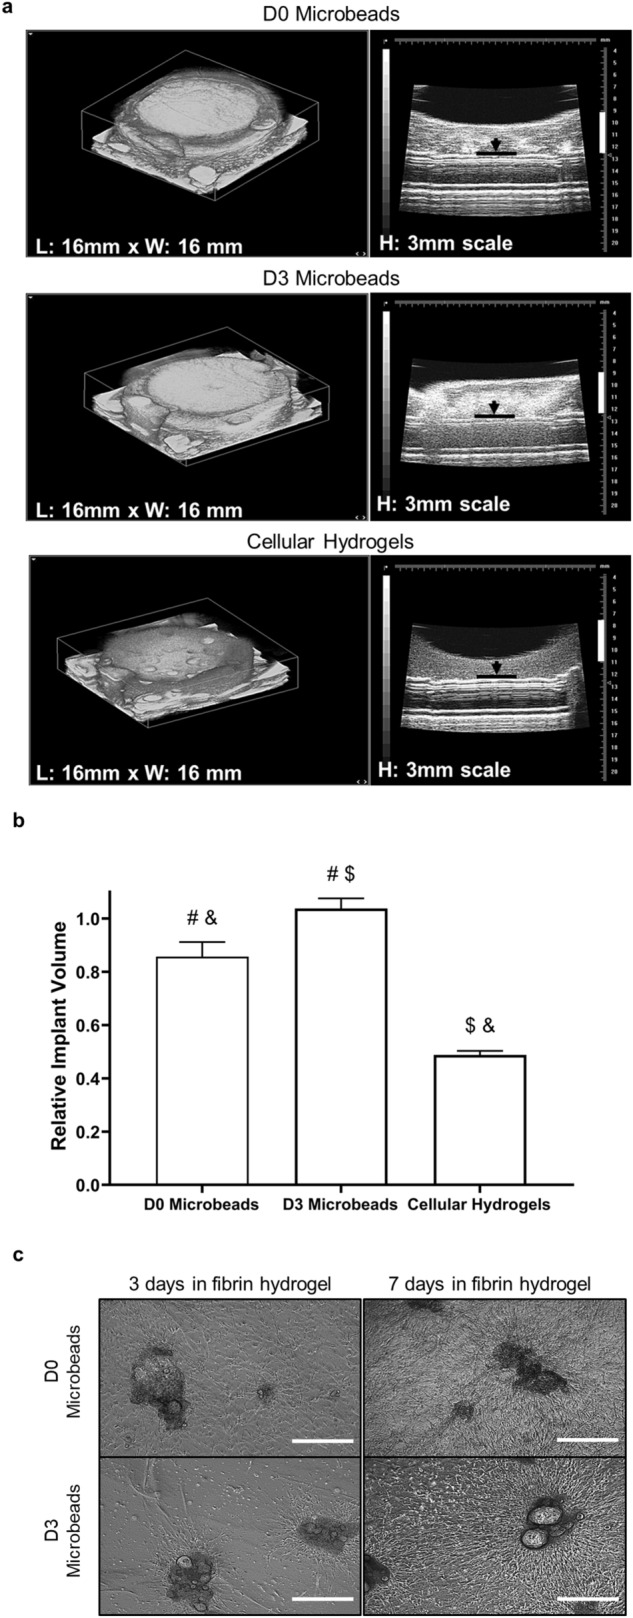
Constructs with D3 microbeads do not compact after 1 day of in vitro culture. (a) 3D ultrasound images of model fibrin implants containing D0 microbeads (top row), D3 microbeads (middle row), or uniformly suspended cells (cellular hydrogels, bottom row). Black lines and arrows in the images on the right-hand side show the bottom of the implant; white vertical lines are 3 mm scale bars. (b) Relative implant volumes were quantified and normalized to the model implants containing D3 microbeads, whose volumes were constant after 24 h of in vitro culture. Statistically significant differences (*p* ≤ 0.05) are indicated by matched symbols. Constructs containing D3 microbeads were of significantly larger volume than those containing D0 microbeads or the cellular hydrogels. (c) Cells deployed more slowly from D3 microbeads when embedded in fibrin hydrogels. Bright-field images of D0 (top row) and D3 (bottom row) microbeads embedded in fibrin hydrogels for 3 (left column) and 7 days (right column). Scale bar = 500 µm. Error bars indicate ± SD.
